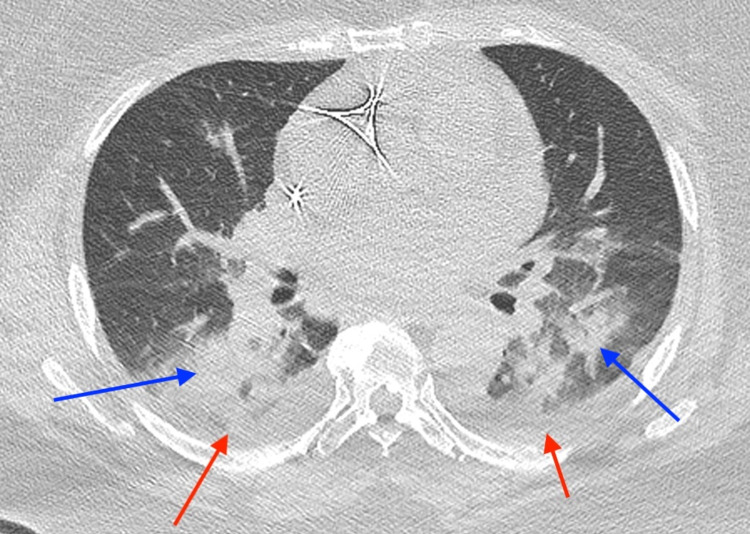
Computed tomography without contrast demonstrating small bilateral pleural effusions and infiltrates Red arrows: demonstrating the bilateral pleural effusions; blue arrows: demonstrating the bilateral infiltrates
